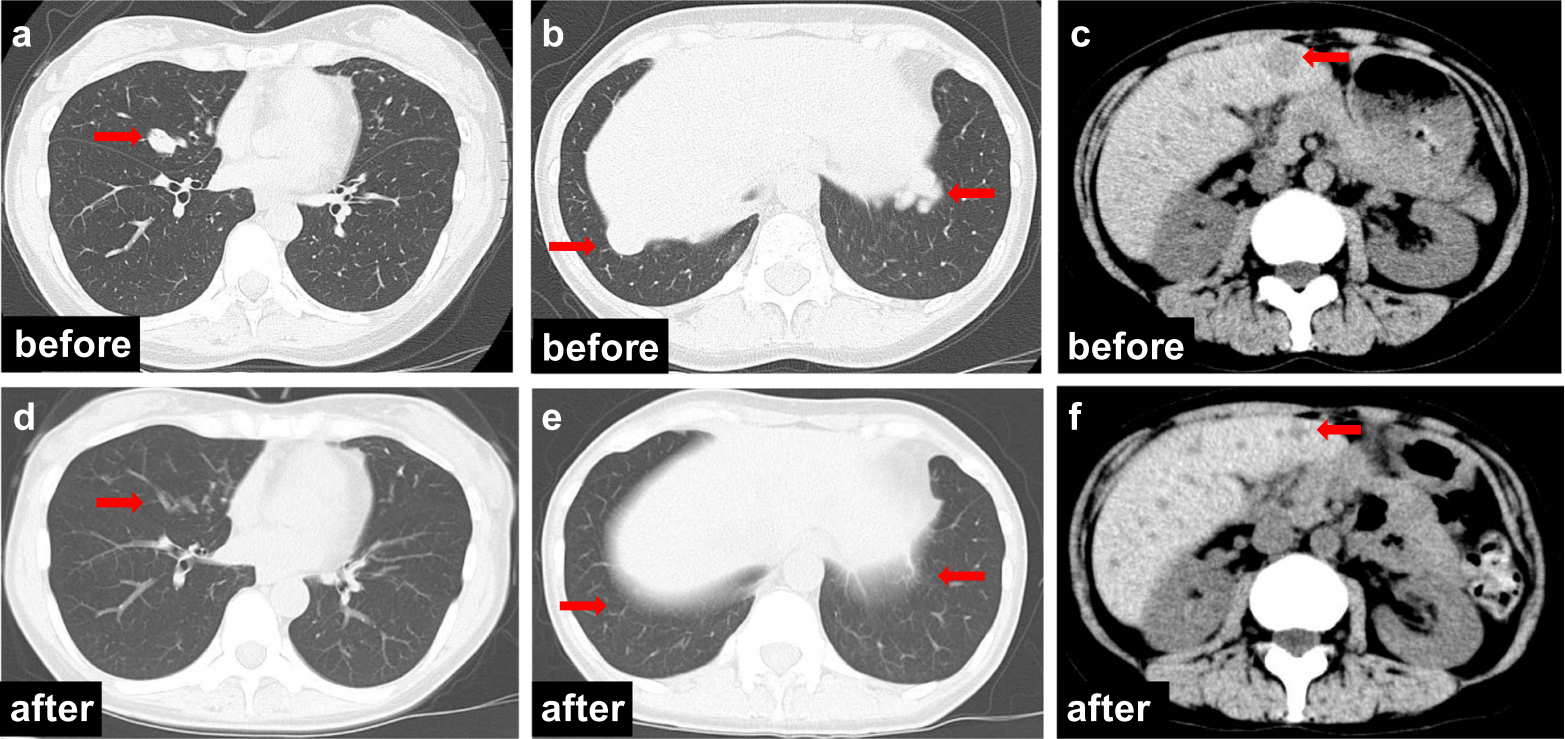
Whole-body CT before and after chemotherapy. Lung metastatic nodules (a, b) and liver tumors (c) were detected. According to treatment with oral 5-FU, the lung metastatic tumors completely disappeared (d, e), and the liver metastases remarkably regressed (f)
